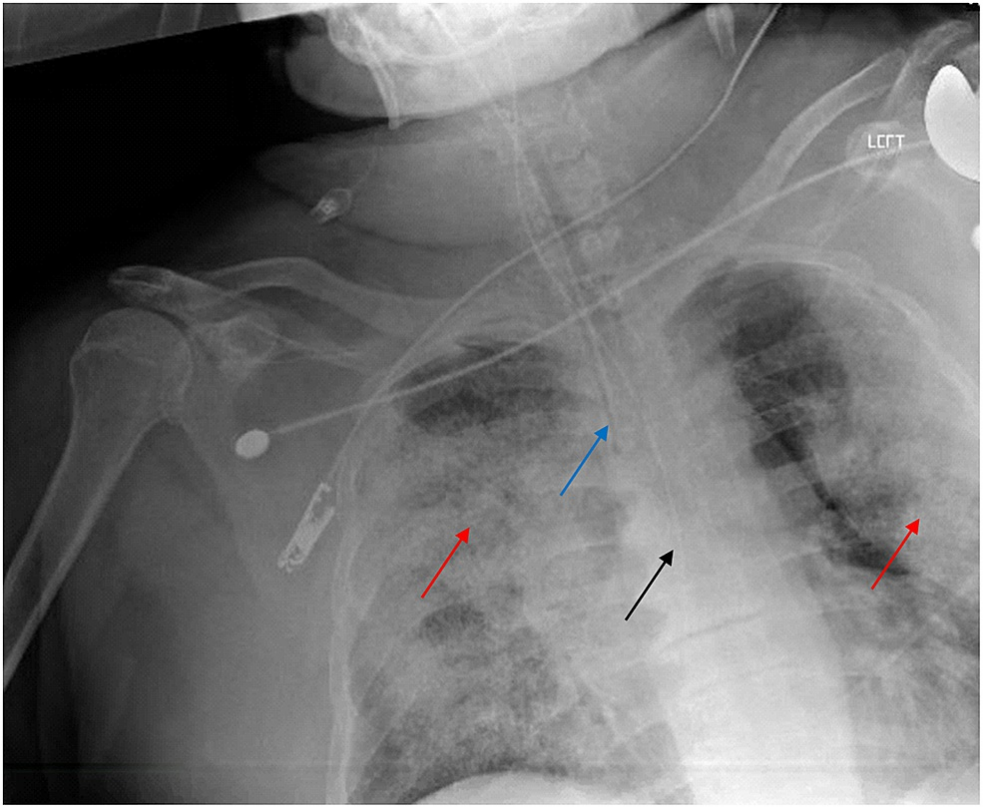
Chest radiograph Chest radiograph showing diffuse bilateral alveolar infiltrates (red arrows). An endotracheal tube terminates above the carina (blue arrow). An orogastric tube extends toward the stomach reaching at least the distal esophagus (black arrow).
